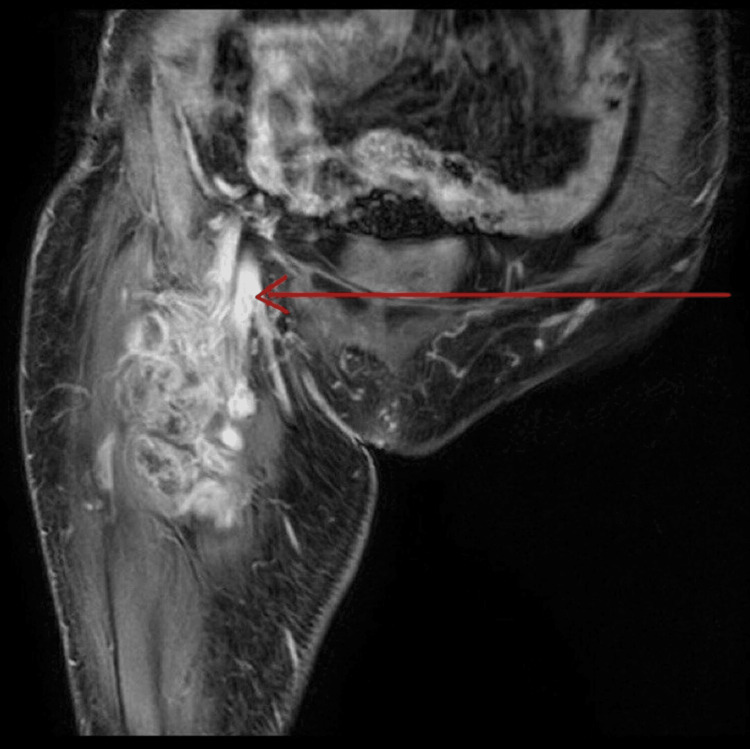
T1-weighted coronal section post-contrast image showing evidence of an altered signal intensity lesion in the intermuscular plane of the right thigh involving the vastus intermedius, vastus medialis, and distal iliopsoas muscles showing moderate post-contrast enhancement. The lesion is encasing deep profunda femoral vessels and femoral nerve.
